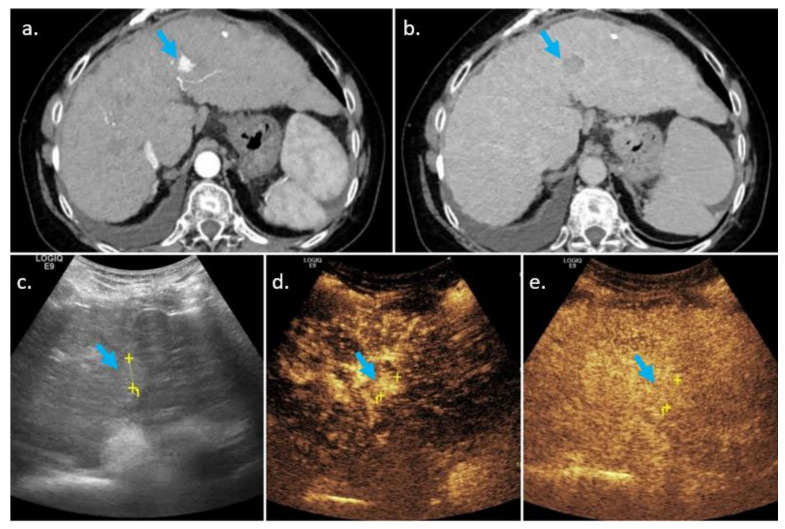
CT scans of the liver obtained in the arterial (a) and late phases (b). A hypervascular nodule (blue arrow) is seen in segment eight of the liver (a). In the late phase (b), the nodule becomes isoenhancing to the liver parenchyma (blue arrow). The nodule was consequently classified as LI-RADS 4 on CT. On US (c), the nodule was slightly hypoechoic/isoechoic (blue arrow) as compared to the surrounding liver parenchyma. On CEUS, the nodule (blue arrow) presented APHE (d) and late and mild washout (e), and was classified as LI-RADS 5.
